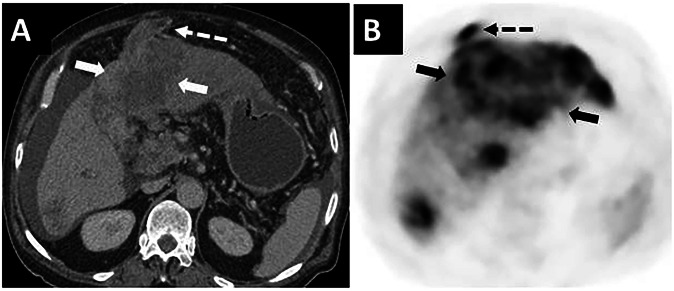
Tumor thrombus in the paraumbilical vein in a patient with known hepatocellular carcinoma. A Axial plane contrast-enhanced CT image and B choline PET-CT image show a diffuse infiltrating lesion in the left liver lobe (arrows) invading the left portal vein. Tumor thrombus in the paraumbilical vein is also noted (dashed arrows)
